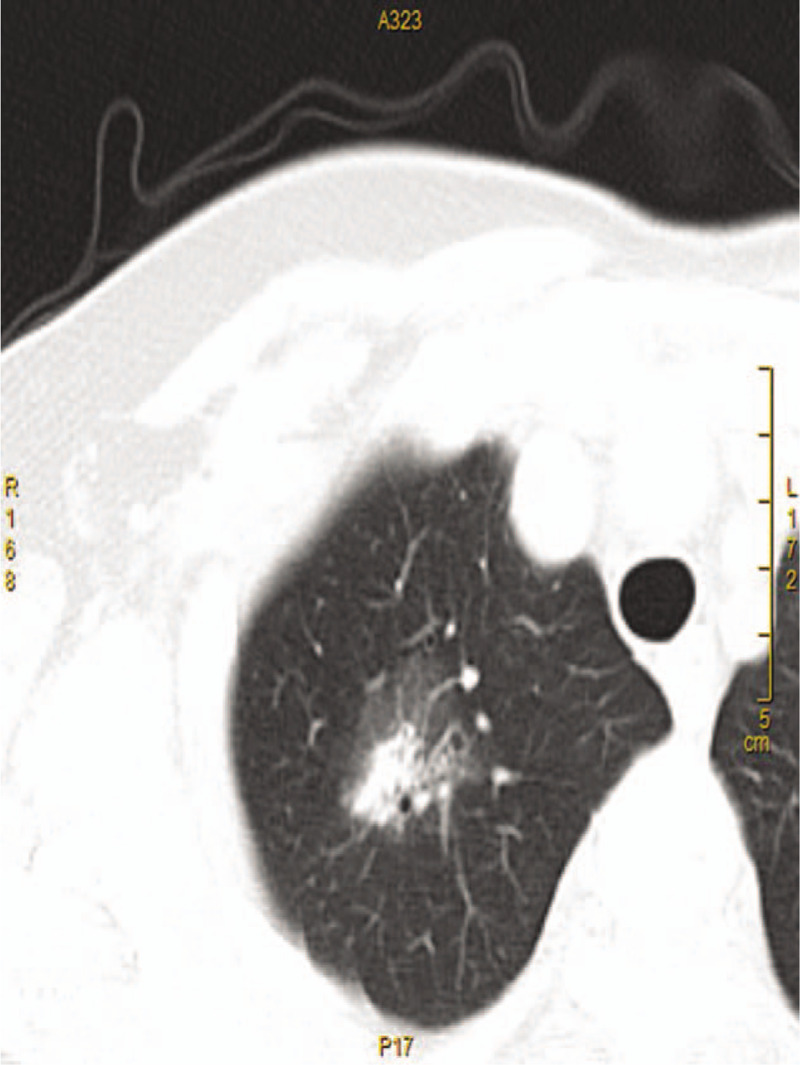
Thoracic computed tomography scan demonstrated a mass (2 cm) in upper lobe of right lung.
